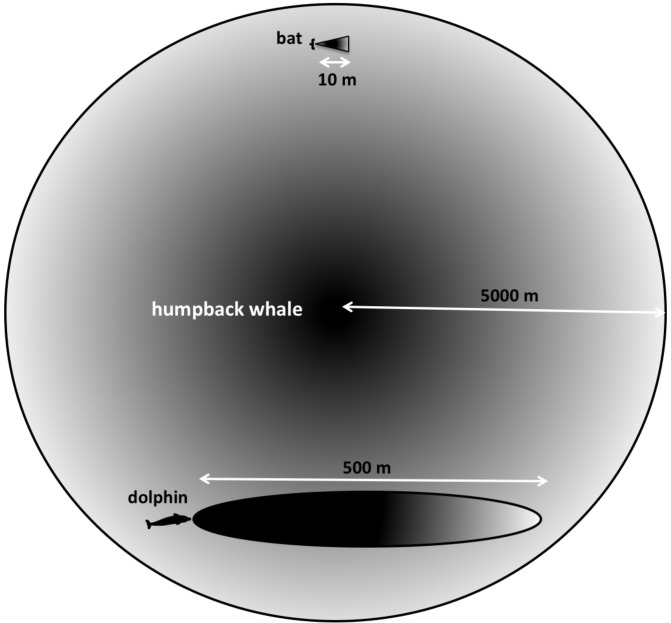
Searching at different scales. “Long-range” for a bat searching for centimeter-sized targets in air is approximately 10 m, whereas dolphins can detect multi-centimeter-sized targets located hundreds of meters away. Because both bats and dolphins use ultrasonic signals, their sonar beams are highly directional. Humpback whales’ lower frequency signals are more omnidirectional, and potentially can enable them to detect multi-meter-sized targets at much farther distances.
